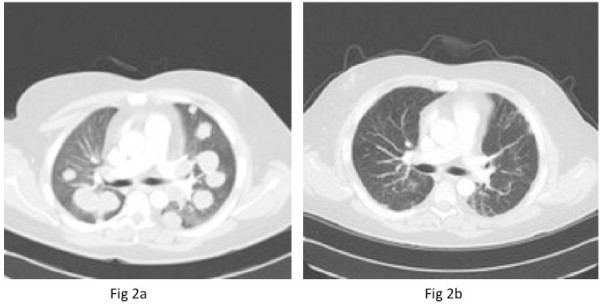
CT Scans: (a) Pre-chemotherapy computed tomography (CT) scan of the thorax demonstrating bilateral widespread pulmonary metastasis. (b) Post-chemotherapy CT scan of the thorax demonstrating resolution of the pulmonary metastasis.
